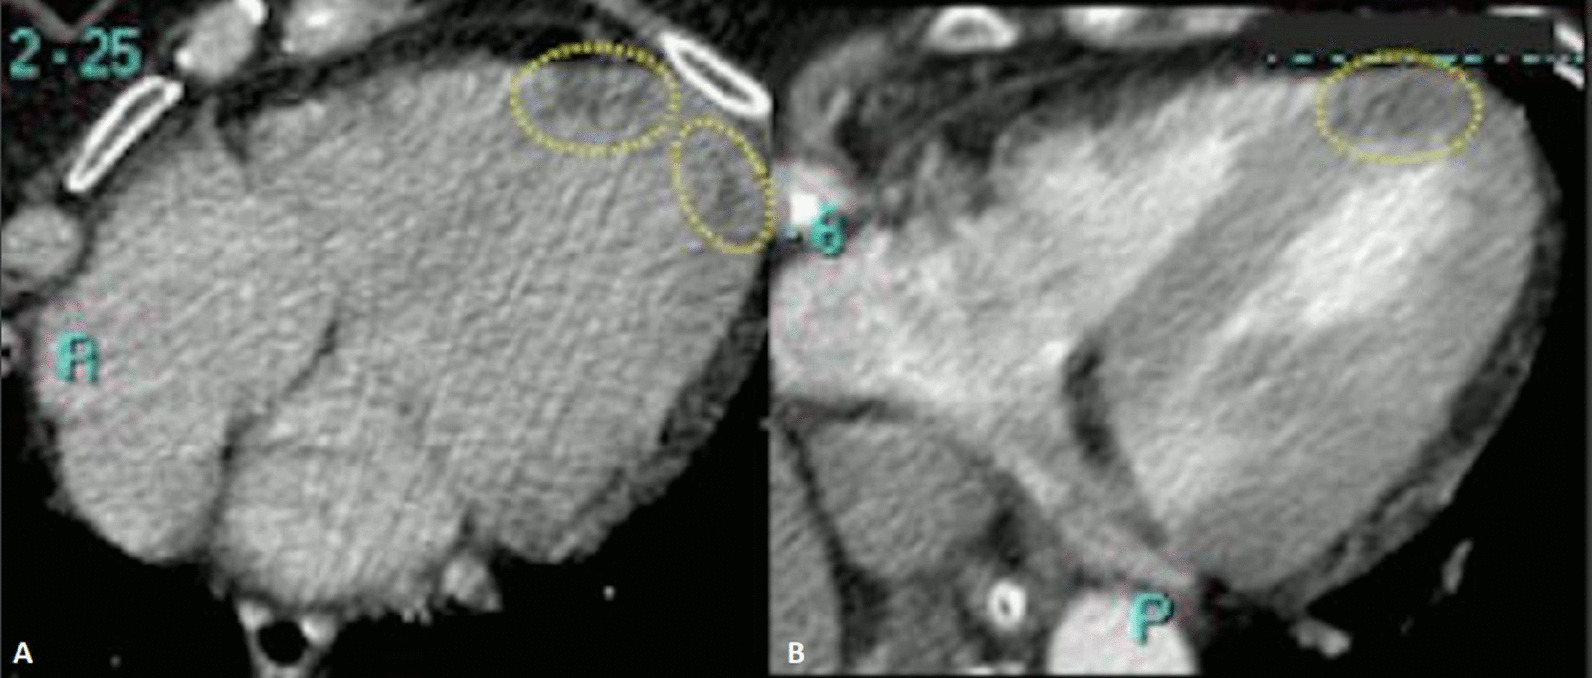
Case 9: Figure 9. Abdominal computed tomography (CT). The heart had been partially covered in two previous abdominal scans related colonic pathology (2016 (A) 2018 (B)). On both scans a focal myocardial hypodensity is visible in the same segments with abnormal signal intensity on CMR. The CT density is similar to the one showed by the pericardial and subcutaneous tissue
